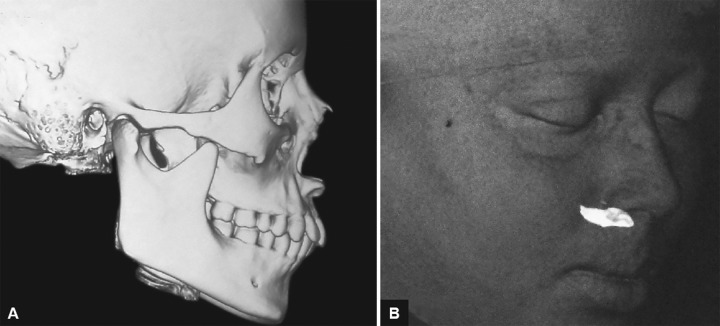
Computed tomography scan shows strange position of maxillary right central incisor and soft tissue recreation on CT scan and strange tooth position
